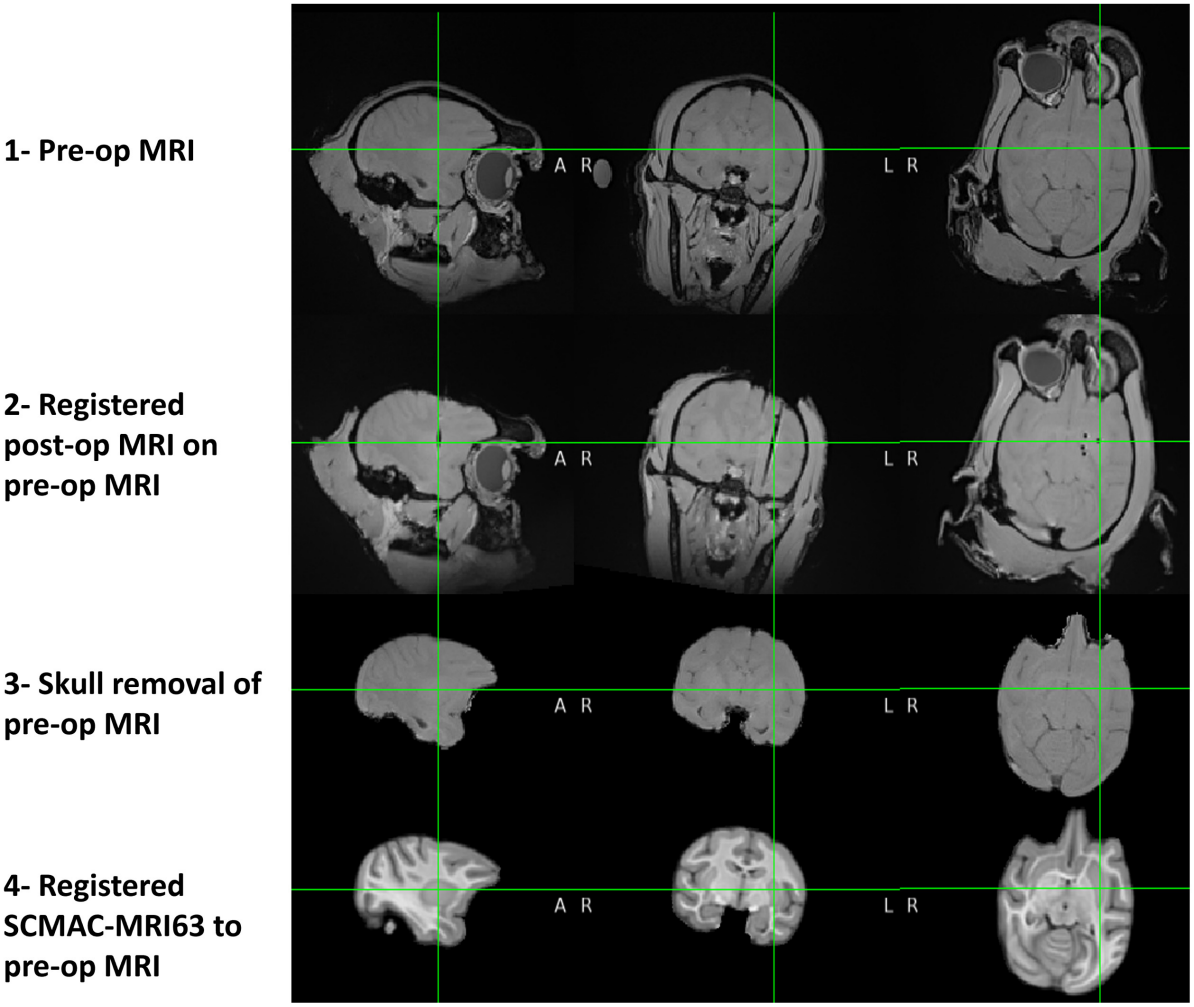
Overview of the skull removal and registration process, aligning the post-op MRI to the pre-op MRI to determine the imaging trajectory coordinates in the pre-op MRI scan, followed by the registration of the SCMAC-MRI63 template to the pre-op MRI from the CERVO-MRI dataset.
